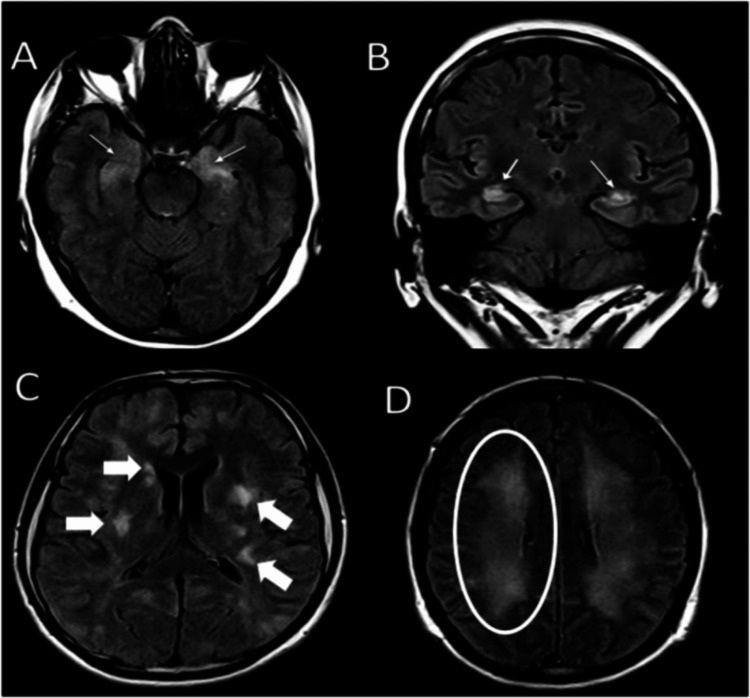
Cranial magnetic resonance imaging of hyperintense lesions MRI fluid-attenuated inversion recovery (FLAIR) sequence. (A and B) Bilateral hippocampal hyperintensities (light arrows). (C) Bilateral asymmetrical multifocal hyperintensities on cortical, subcortical, and periventricular zones (arrows). (D) Diffusive bilateral lesions on frontal lobes white matter (circle).
